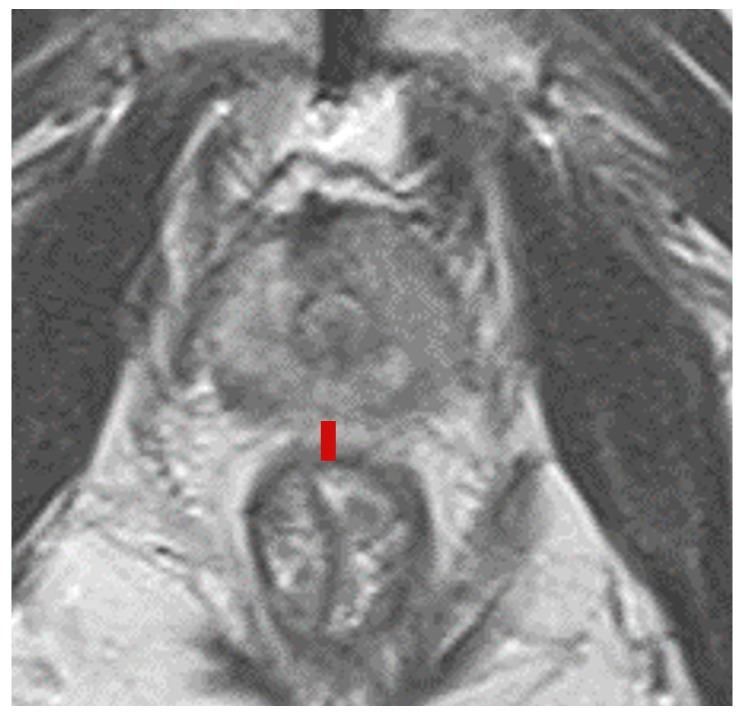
The distance from the prostate capsule to the rectal mucosa (DPR) was measured using horizontal MRI images obtained before prostate biopsy or treatment. DPR was measured at three locations: the apex, midpoint, and base of the prostate. The median value of the DPR measured at the midpoint was defined as the mDPR. The red line indicates the DPR.
